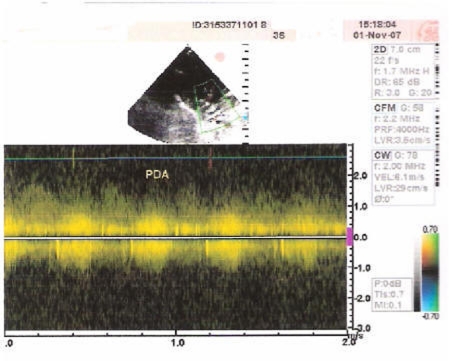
Echocardiogram showing PDA with continuous blood flow.
